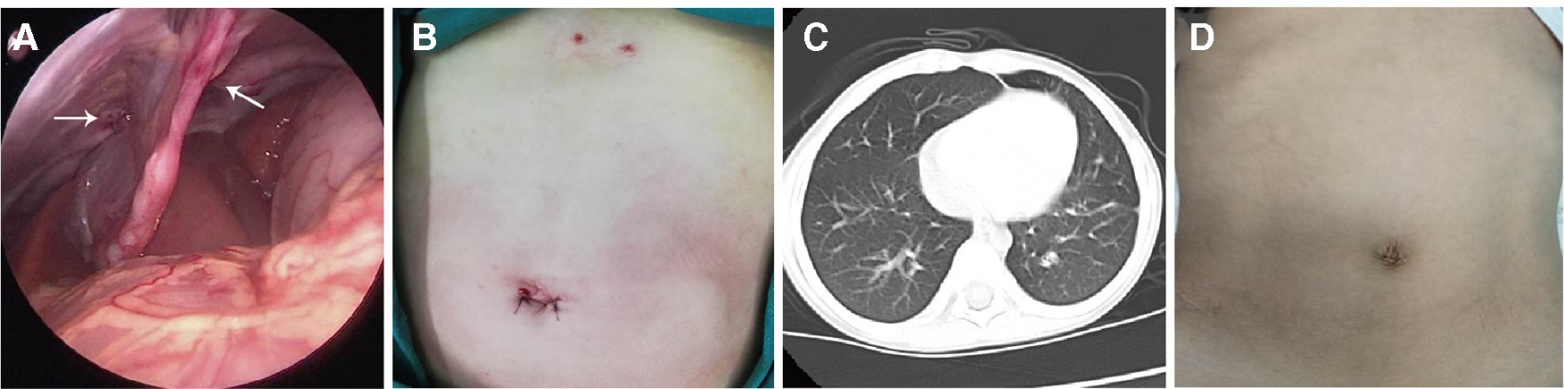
Postoperative outcomes. (A) Ligation of the bilateral hernia sac: ligation was firm and did not leave any gaps; (B) Abdominal incisions: the umbilical incision was small, and the extracorporeal knots were located under the xiphoid process; (C) Chest CT 15 months after the operation: the shadow behind the sternum had disappeared, and there was no aggravation of the pectus carinatum; (D) Aesthetic outcomes were satisfactory 15 months after the operation.
